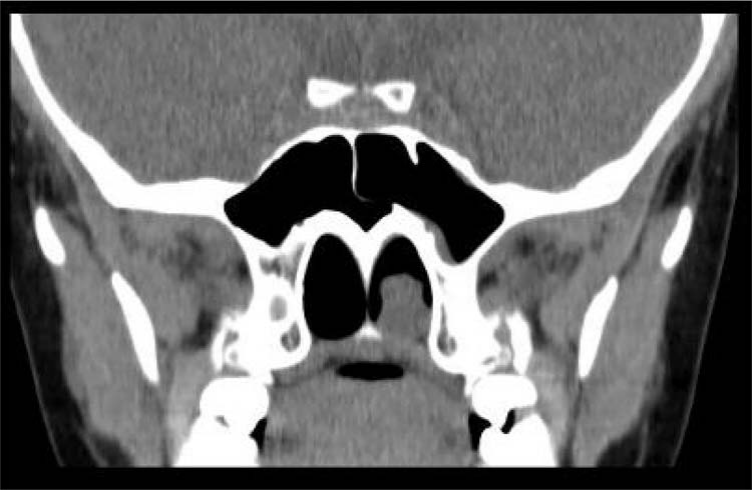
CT scan—coronal cut showing a homogenous mass occupying the inferior aspect of the left choana.
